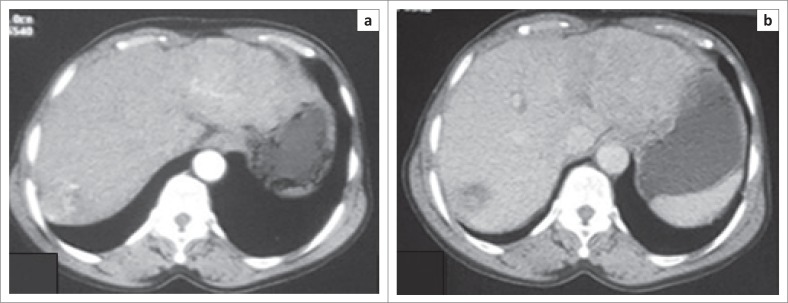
A 54-year-old male with hepatocellular carcinoma in segment VII of the liver who presented with right upper-quadrant pain. Axial computed tomography scans showing: (a) arterial phase enhancement and (b) rapid washout in the portovenous phase.
